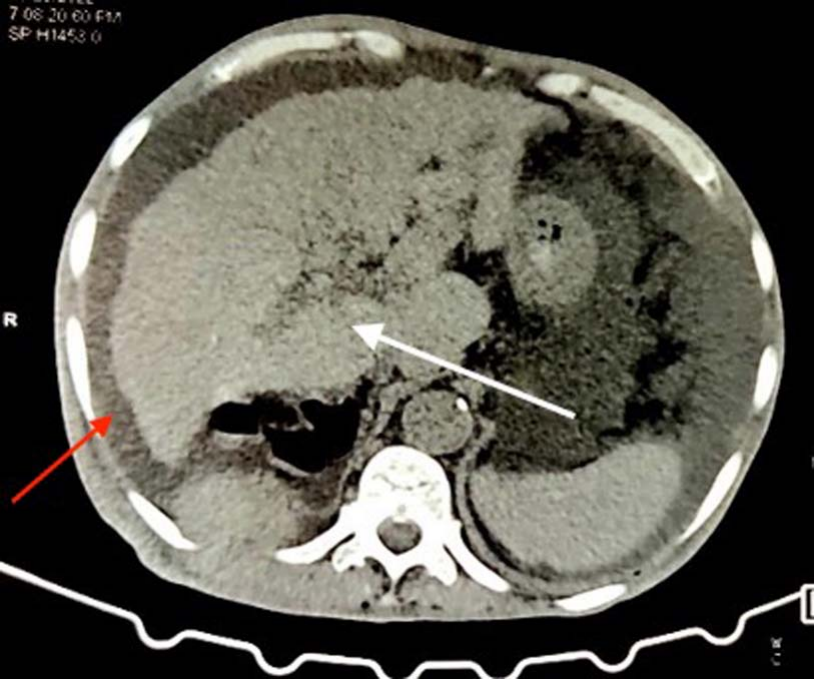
Axial contrast-enhanced abdomen CT shows diffuse irregularity of liver (white arrow) and ascites (red arrow). CT, computed tomography.
